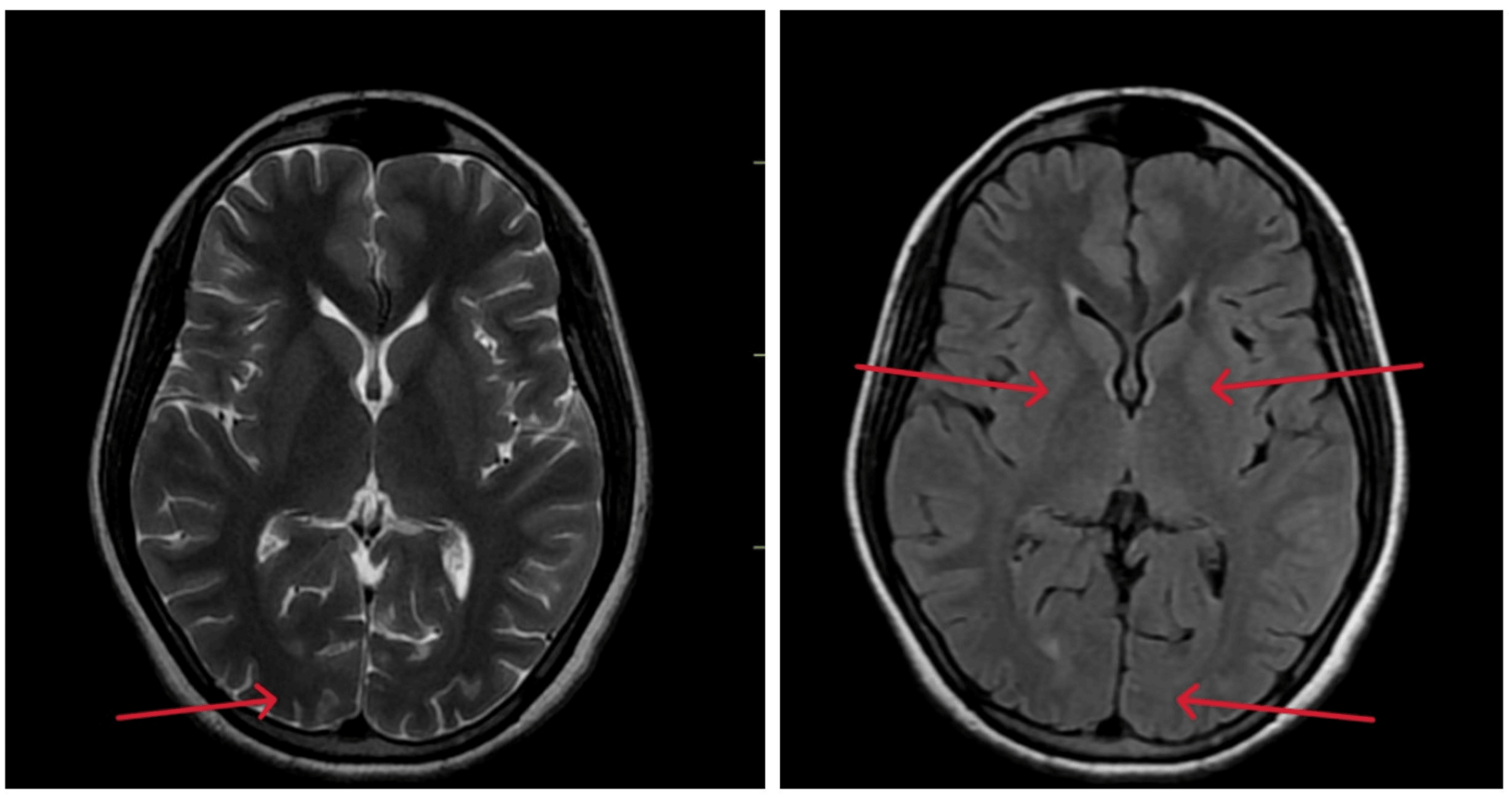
Follow-up MRI of the brain (axial section), five days later, showing significant improvement in the pathology, with no significant lesions observed in the same region as before (red arrow).
